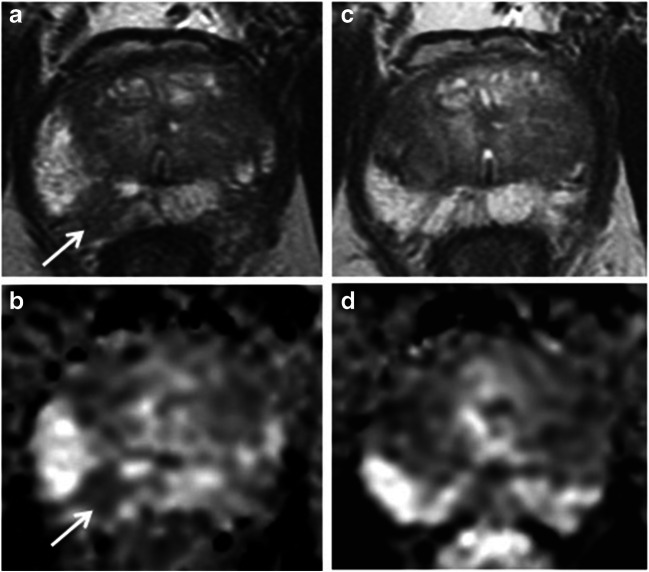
PRECISE score 1. A 62-year-old patient at enrolment, with PSA of 4.53 ng/ml. Top row: T2 axial; bottom row: ADC maps. a, b MRI shows Likert 3 lesion at the right apex PZ (arrows), with mild-to-moderate restricted diffusion. Targeted biopsy shows Gleason 3 + 3 = 6 in 1/2 cores, 2 mm, 5%. c, d MRI at 24 months shows almost a complete resolution of T2 intermediate signal intensity change (c) and no restricted diffusion on ADC (d)
